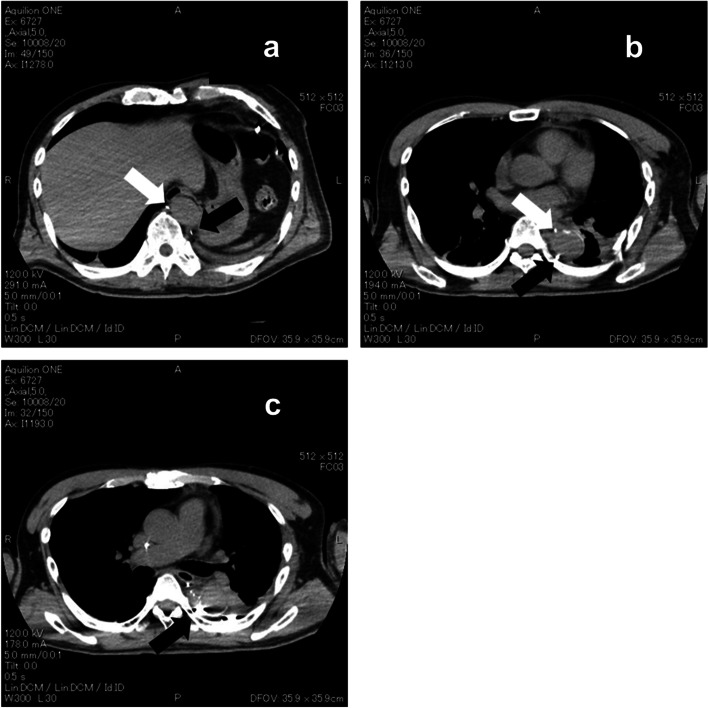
Chest computed tomography during lymphangiography. a, b Two thoracic ducts were observed (white arrow, black arrow). No connections were observed. c Leakage site from the thoracic duct running through the left thoracic cavity (black arrow)
